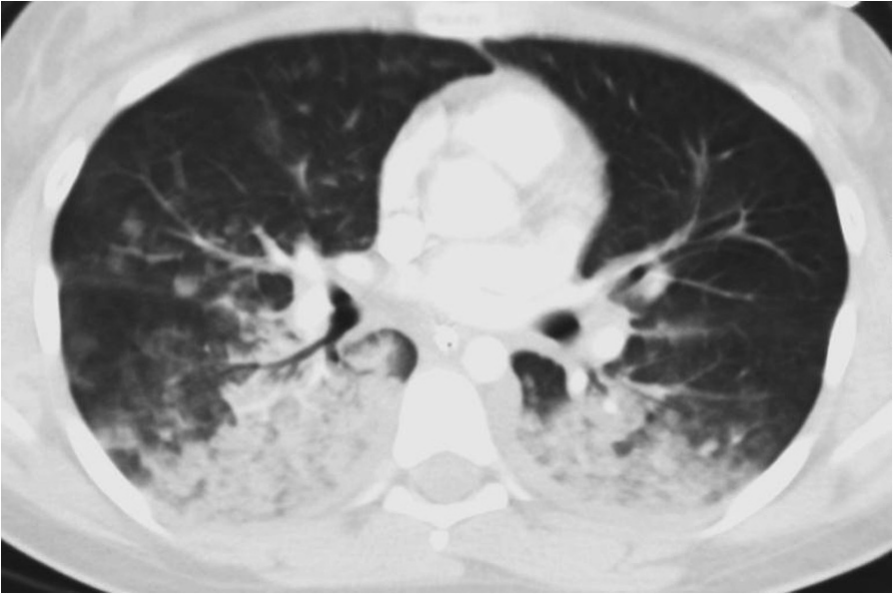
CT scan of same patient several hours after admission. Patient had undergone craniotomy for evacuation of intracranial hemorrhage. Intracranial pressure was not elevated post-operatively. (Atelectasis and consolidation is heterogeneous at dependent bases).
